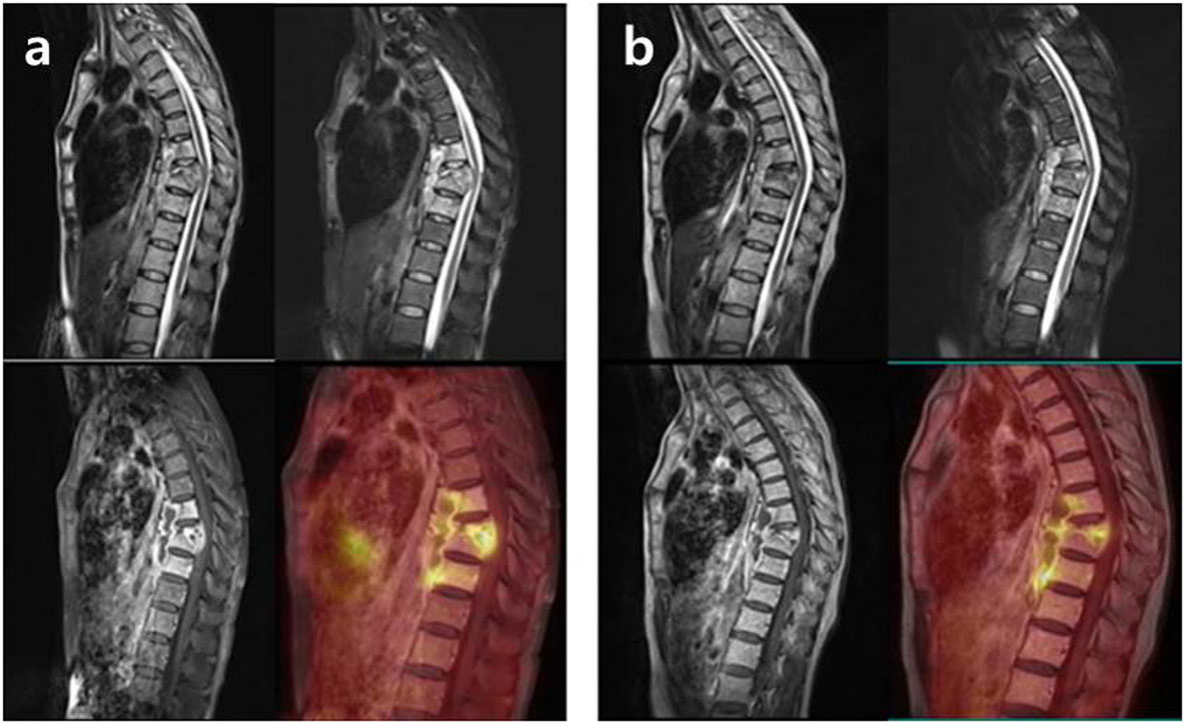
The third patient’s follow-up PET/MRI at 12-month anti-TB medication therapy (b) revealed sustained abscesses around the paravertebral space with intra-abscess SUV_max_ (from 6.67 of PET/MRI at diagnosis to 7.02) even though decreased bone marrow edema and epidural abscess on T8–9 compared with PET/MRI at diagnosis (a). After additional three months of anti-TB medication, the leak of the tuberculous abscess through the fistula developed again
